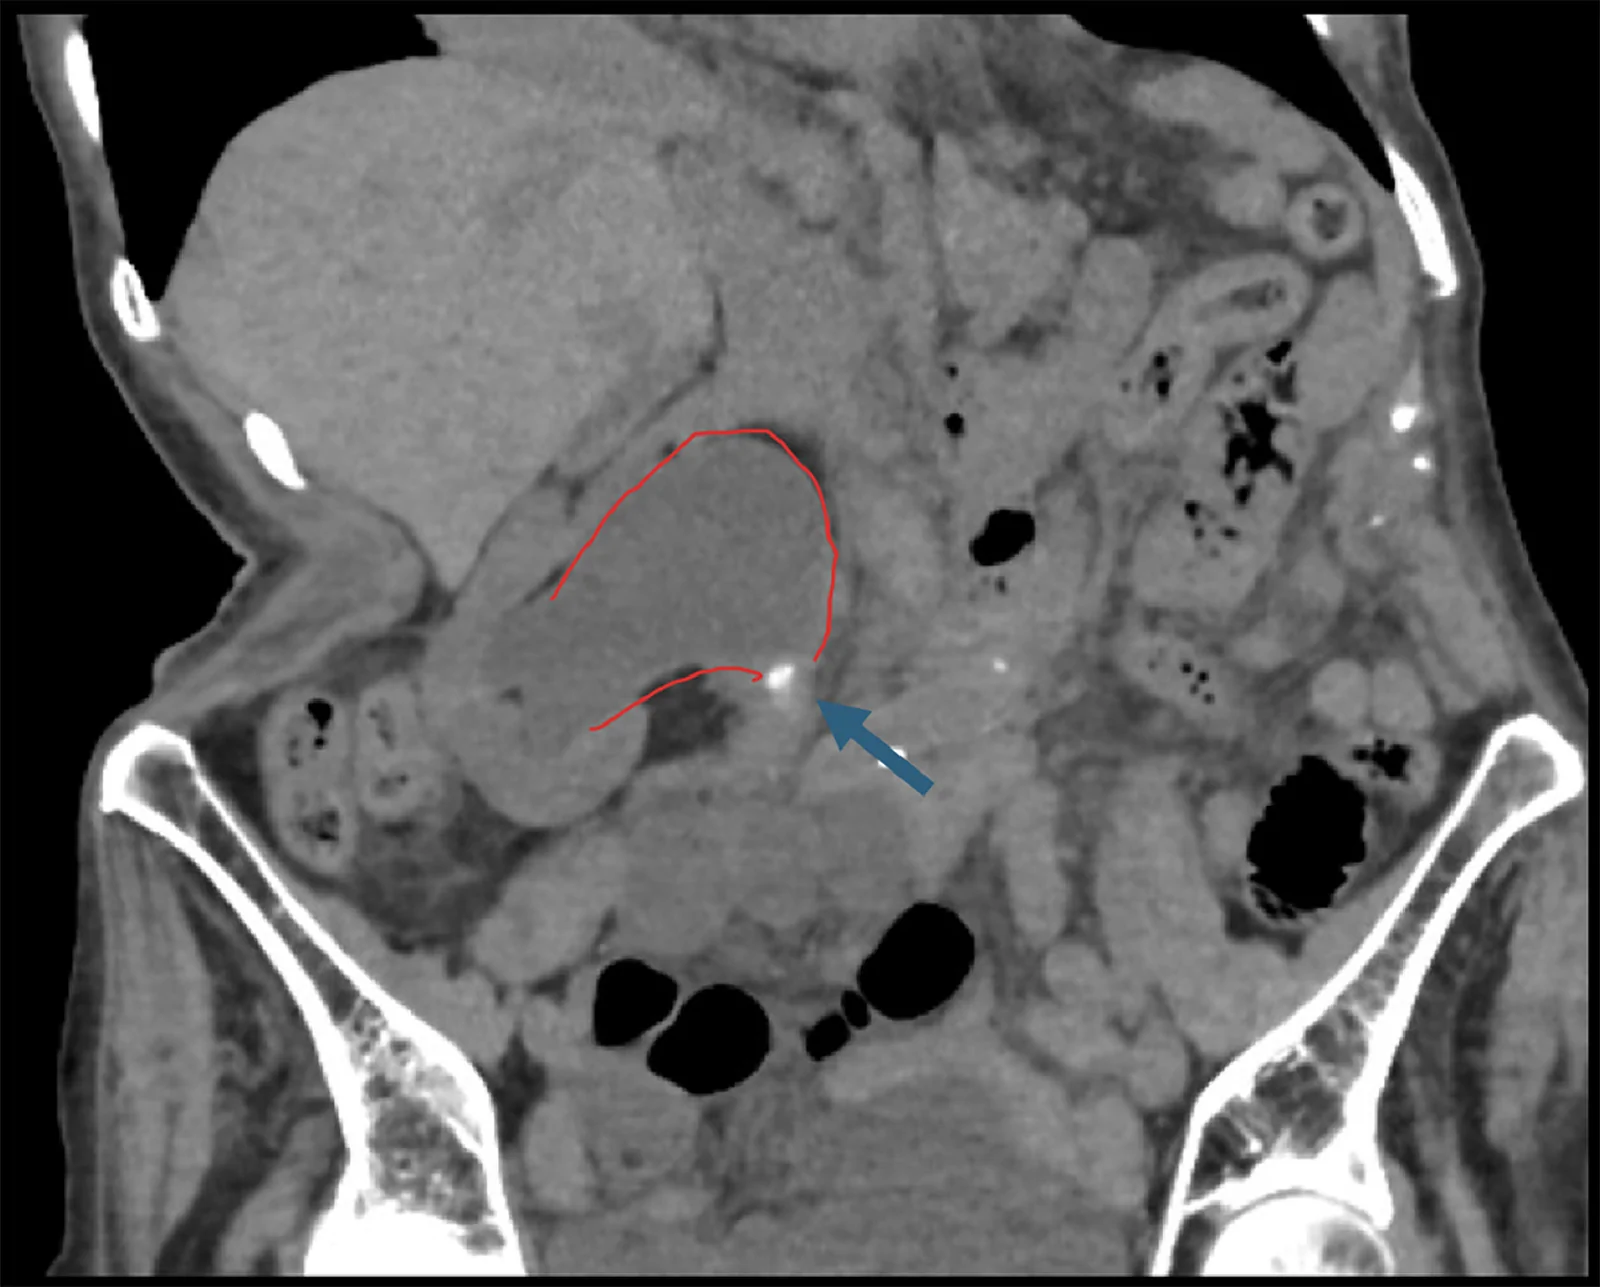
Coronal noncontrast CT image demonstrates sided hydronephrosis (red outline) secondary to a stone at the uteropelvic junction. Several nonobstructed loops of bowel are noted as well.
